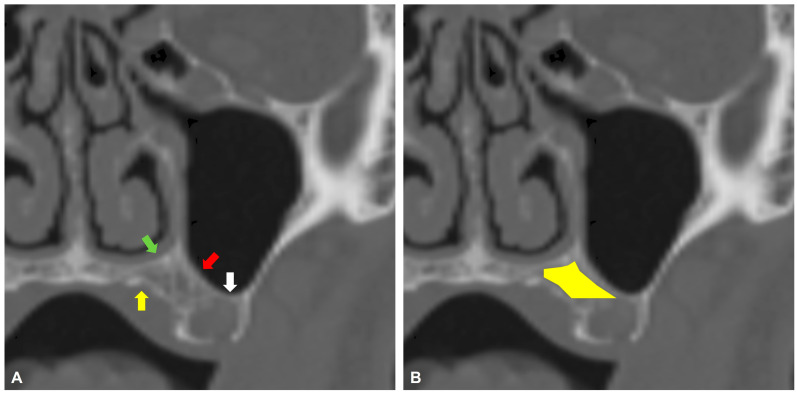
Helical CT coronal view demonstrating the anatomical borders of the palatal bone reservoir. (A) The most inferior point of the maxillary sinus floor (white arrow), the medial part of the maxillary sinus floor (red arrow), the horizontal palate (yellow arrow), and the nasal floor (green arrow). (B) The area of the palatal bone reservoir is depicted by a yellow polygon.
